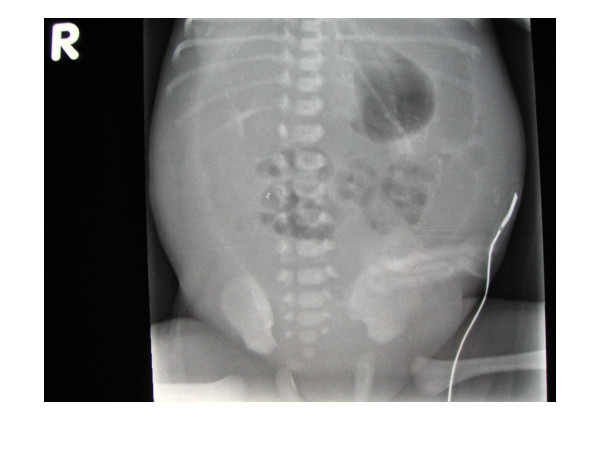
Abdominal radiograph taken 4 hours after birth demonstrating free gas in the abdomen. The infant had not been artificially ventilated at any point pre-operatively.
